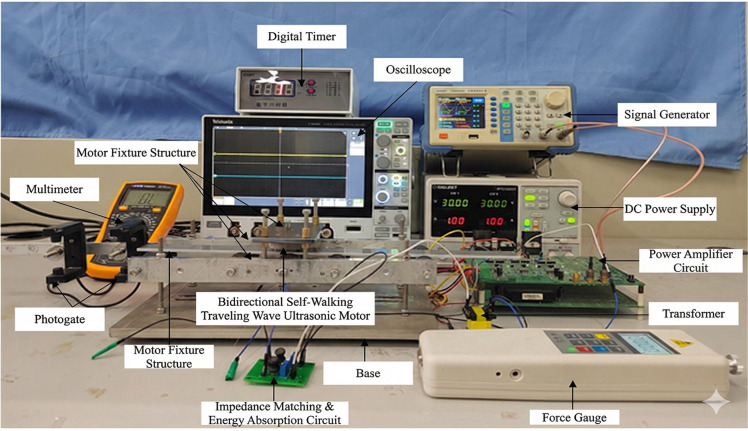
Experimental Platform of a Bidirectional Self-Propelled Traveling-Wave Linear Ultrasonic Motor.
